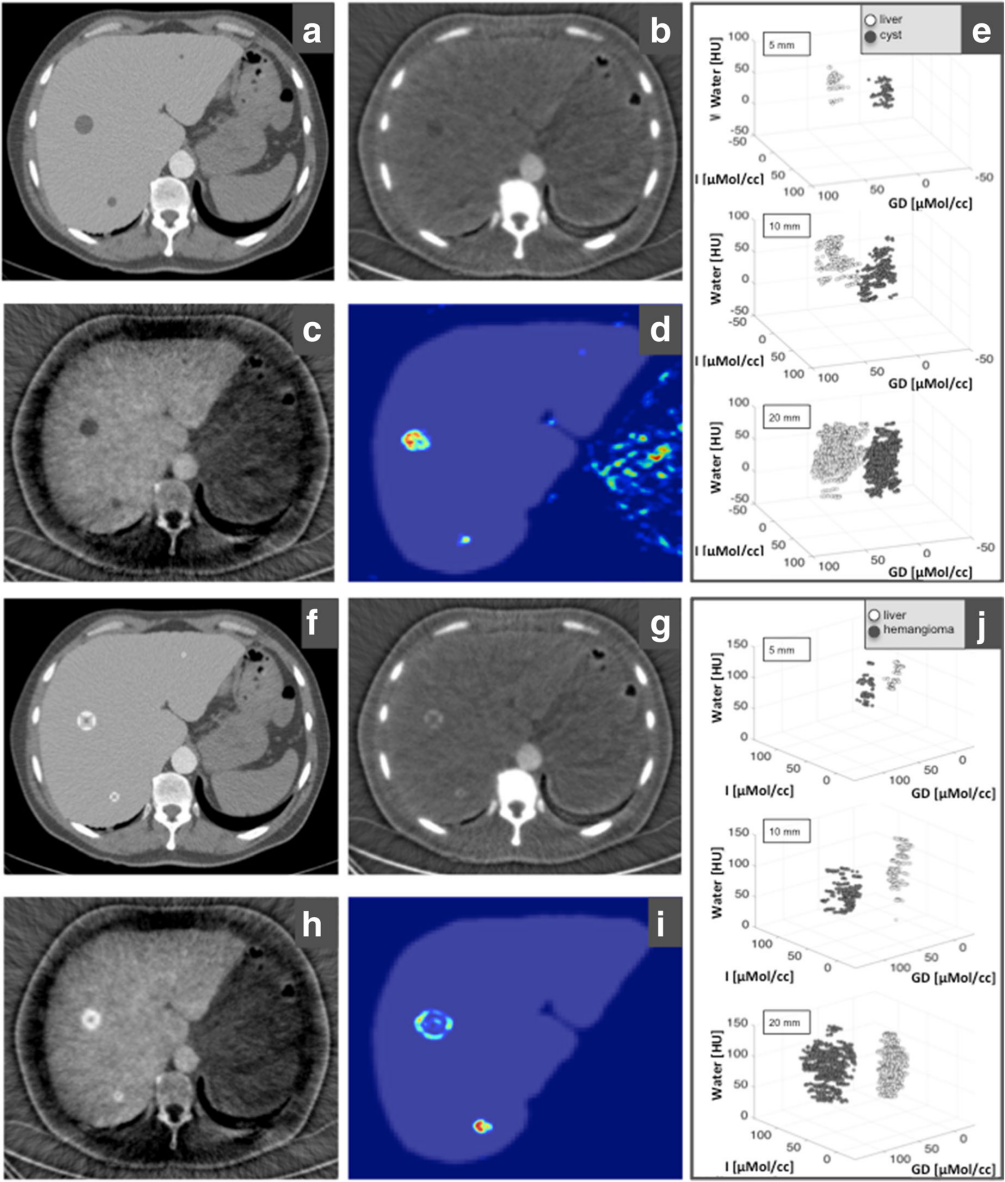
SPCCT imaging of benign liver lesions, exemplified by cysts (a–e) and haemangiomas (f–j). a, f Conventional CT image. b Iodine-enhanced image, showing cysts without any contrast uptake in the arterial phase. c Gadolinium-enhanced image showing cysts in portal venous phase. g, h Iodine- and gadolinium-enhanced image of haemangiomas, with a typical closing-iris pattern. d Likelihood map showing the 20-mm, 10-mm, and 5-mm diameter cysts. i For haemangiomas, the smallest lesion was missed in the calculated likelihood map. e, j
